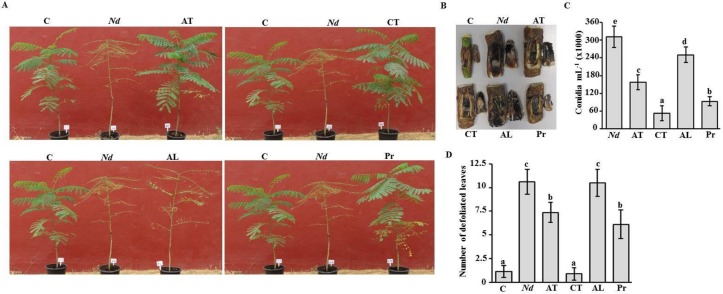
Effect of fungicide treatments on artificially inoculated royal poinciana seedlings with *Neoscytalidium dimidiatum* in the greenhouse. Fungicidal suppression of stem canker disease on royal poinciana seedlings using (A) potential chemical fungicides; (B) symptoms of inoculated regions; (C) number of conidia after recovery of the pathogen from stem tissues; and (D) number of defoliated leaves in inoculated seedlings sprayed with chemical fungicides at 4 wpt. In (A–D), seedlings were inoculated for 2 weeks with *N. dimidiatum* before the fungicide treatment. In (C & D), mean values with different letters are significantly different from each other at *p* < 0.05. Experiments were repeated at least three times with similar results. C, control (no *N. dimidiatum*); *Nd*, *N. dimidiatum*; AT, Amistar Top^®^: CT, Cidely^®^ Top; AL, Airone Liquido^®^, Pr, Protifert^®^; wpt, weeks post treatment.
